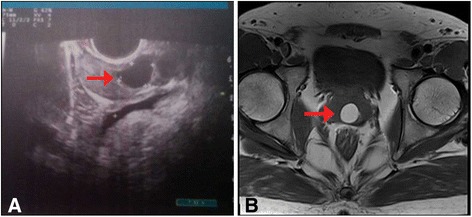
The representative images of MPC. A, TRUS; B, pelvic MRI. The arrow indicates the cystic lesion.
